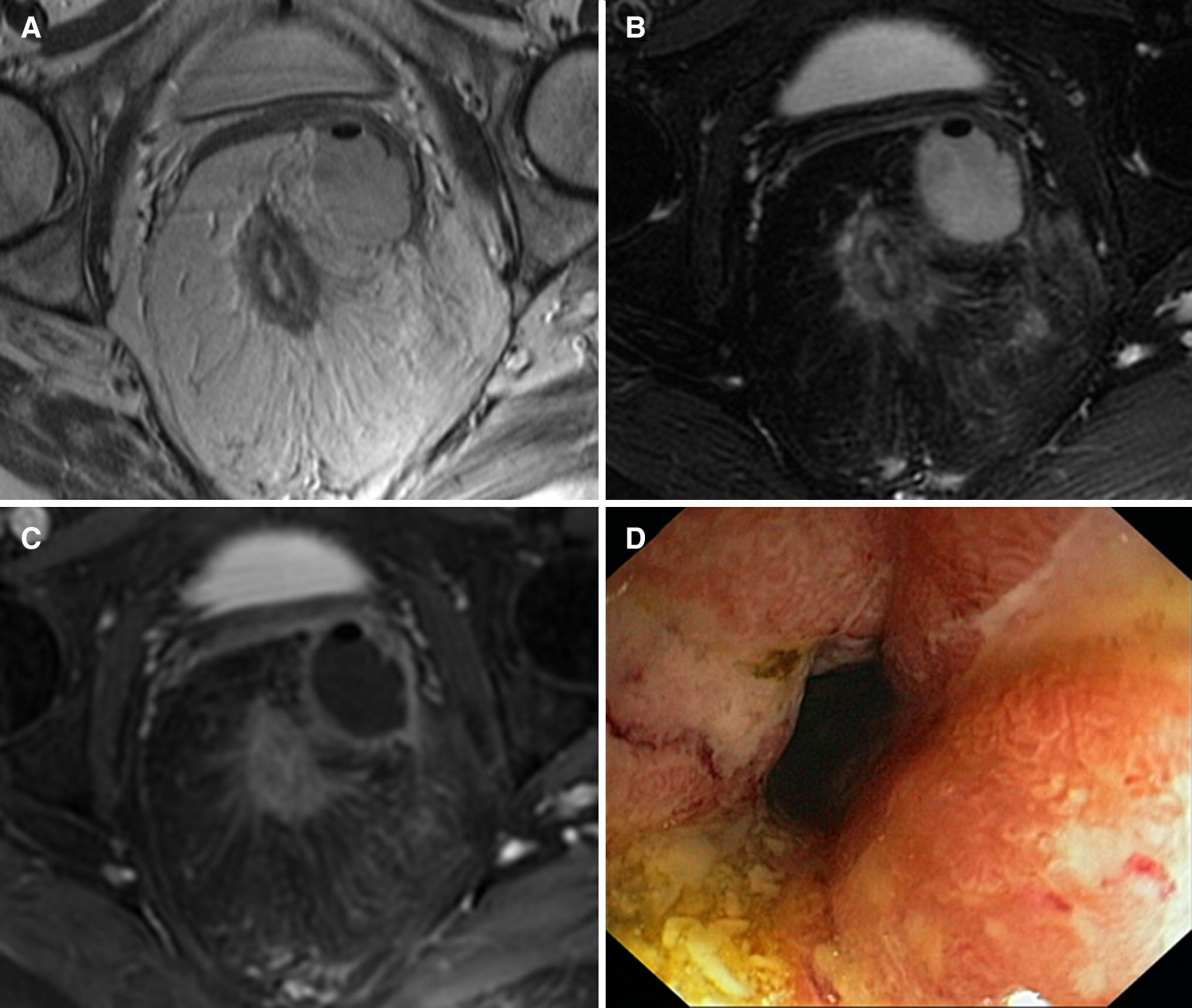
A 49-year-old female with Crohn’s disease. A Axial oblique T2-weighted image and B axial oblique fat-saturated T2-weighted image show rectal wall thickening, a marked increase of T2 signal intensity and a perimural large fluid rim (>2 mm). C Axial oblique fat-saturated post-contrast T1-weighted images obtained at the same level shows a moderate enhancement of the rectal wall and the perimural fat tissue. In addition, there is creeping fat and a supralevatoric abscess left anterolateral of the rectum on all three images. D Endoscopy showed ulcerative proctitis
